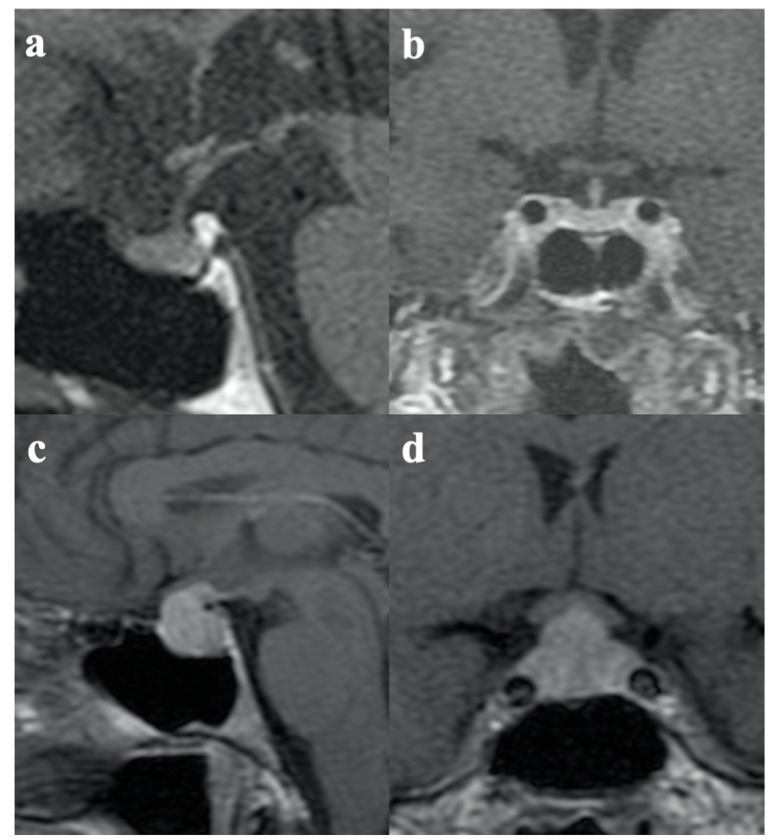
(a) Sagittal and (b) coronal T1-weighted images after contrast administration show normal pituitary and stalk volume and regular enhancement in a patient with ICI-induced hypoadrenalism. (c) Sagittal and (d) coronal T1-weighted images after contrast administration show typical features of hypophysitis: an enlarged pituitary gland and a thickened pituitary stalk with homogenous enhancement in a patient with IIH.
